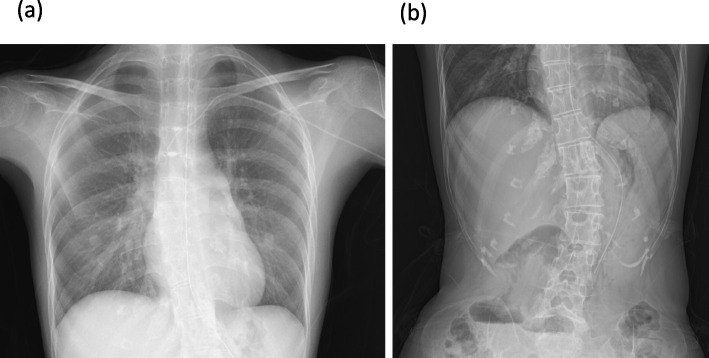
Preoperative radiographs of a chest and b abdomen. There was scoliosis of the thoracolumbar spine and whole-body muscle atrophy, but no severe thoracic deformity
